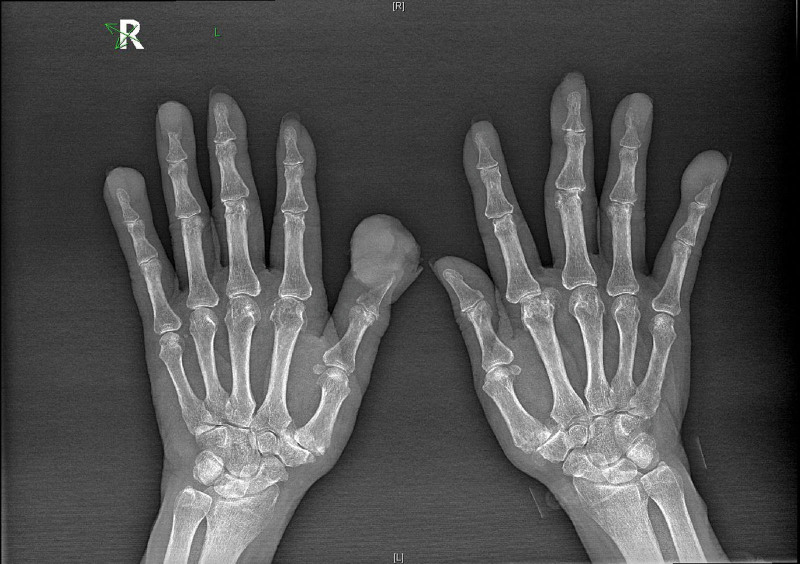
X-rays of both hands before finger resection showing metastatic lesions.
